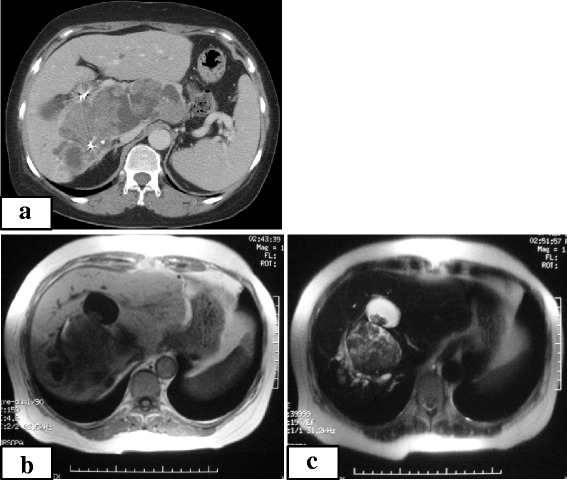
Abdominal contrast computed tomography (post-transcatheter arterial embolization [TAE]) and magnetic resonance imaging findings. (a) Computed tomography scan showed a low-density hepatic lesion measuring 15 cm in diameter with mural nodules. These nodules were contrast-enhanced. The TAE coils were observed. (b) T1-weighted image showed a low-intensity mass, except for the mural nodules. (c) T2-weighted image demonstrated high signals from the mural nodules.
